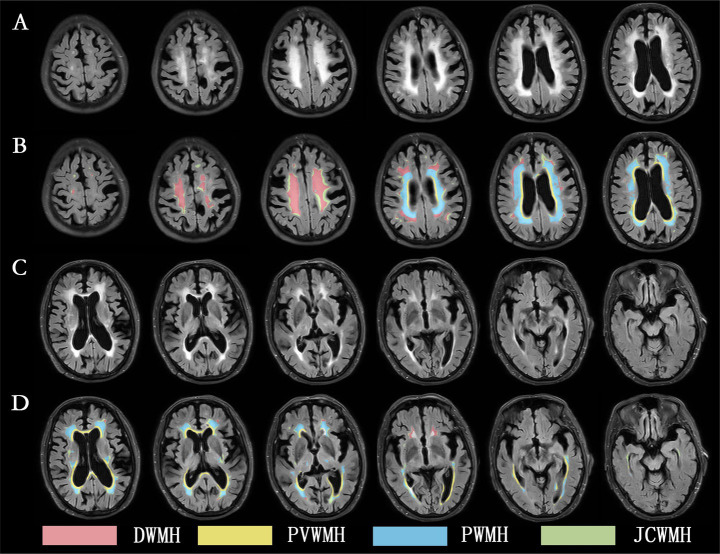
T2-FLAIR image and corresponding processed image. (A,C) T2-FLAIR image; (B,D) corresponding processed image.
